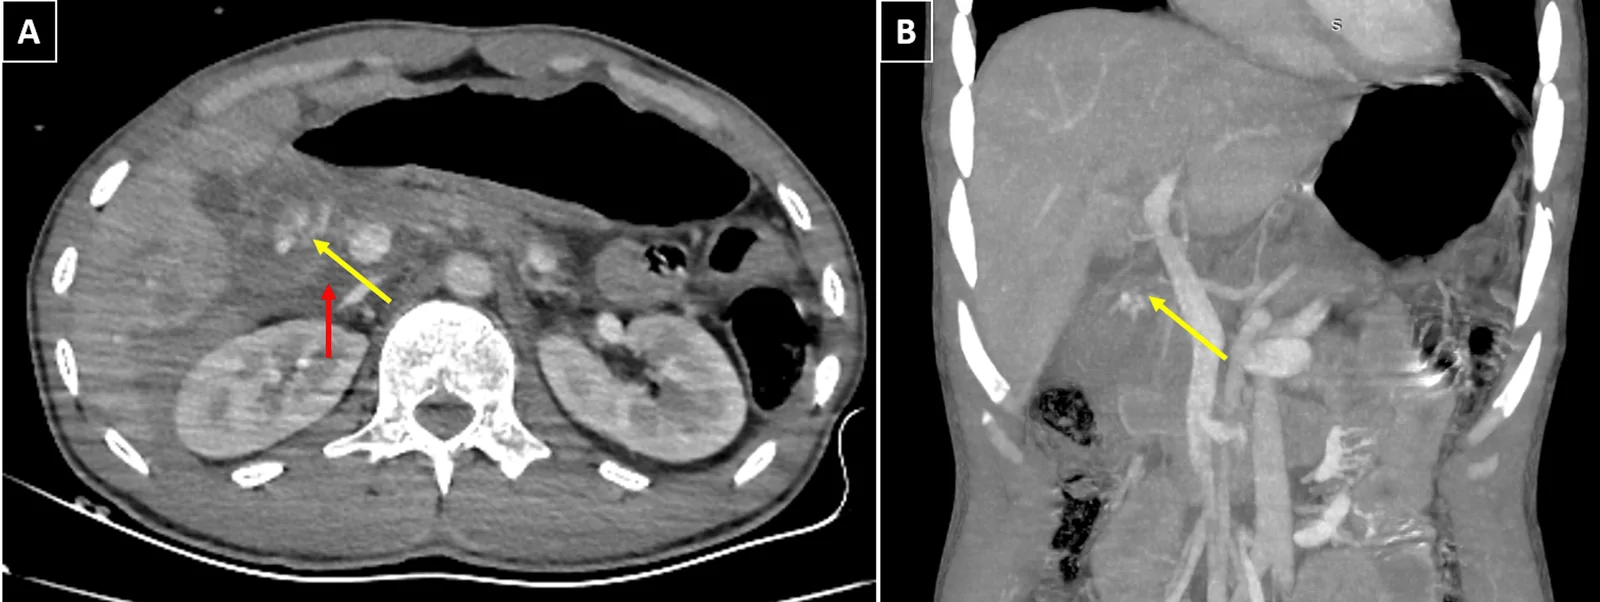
Imaging findings from abdominal post-contrast CT (arterial phase) A: Axial abdominal CT post IV contrast at arterial phase shows contrast endoluminal extravasation (yellow arrow) and adjacent free fluid (red arrow); B: Maximum Intensity Projection (MIP) of coronal reconstruction shows contrast endoluminal extravasation (yellow arrow).
